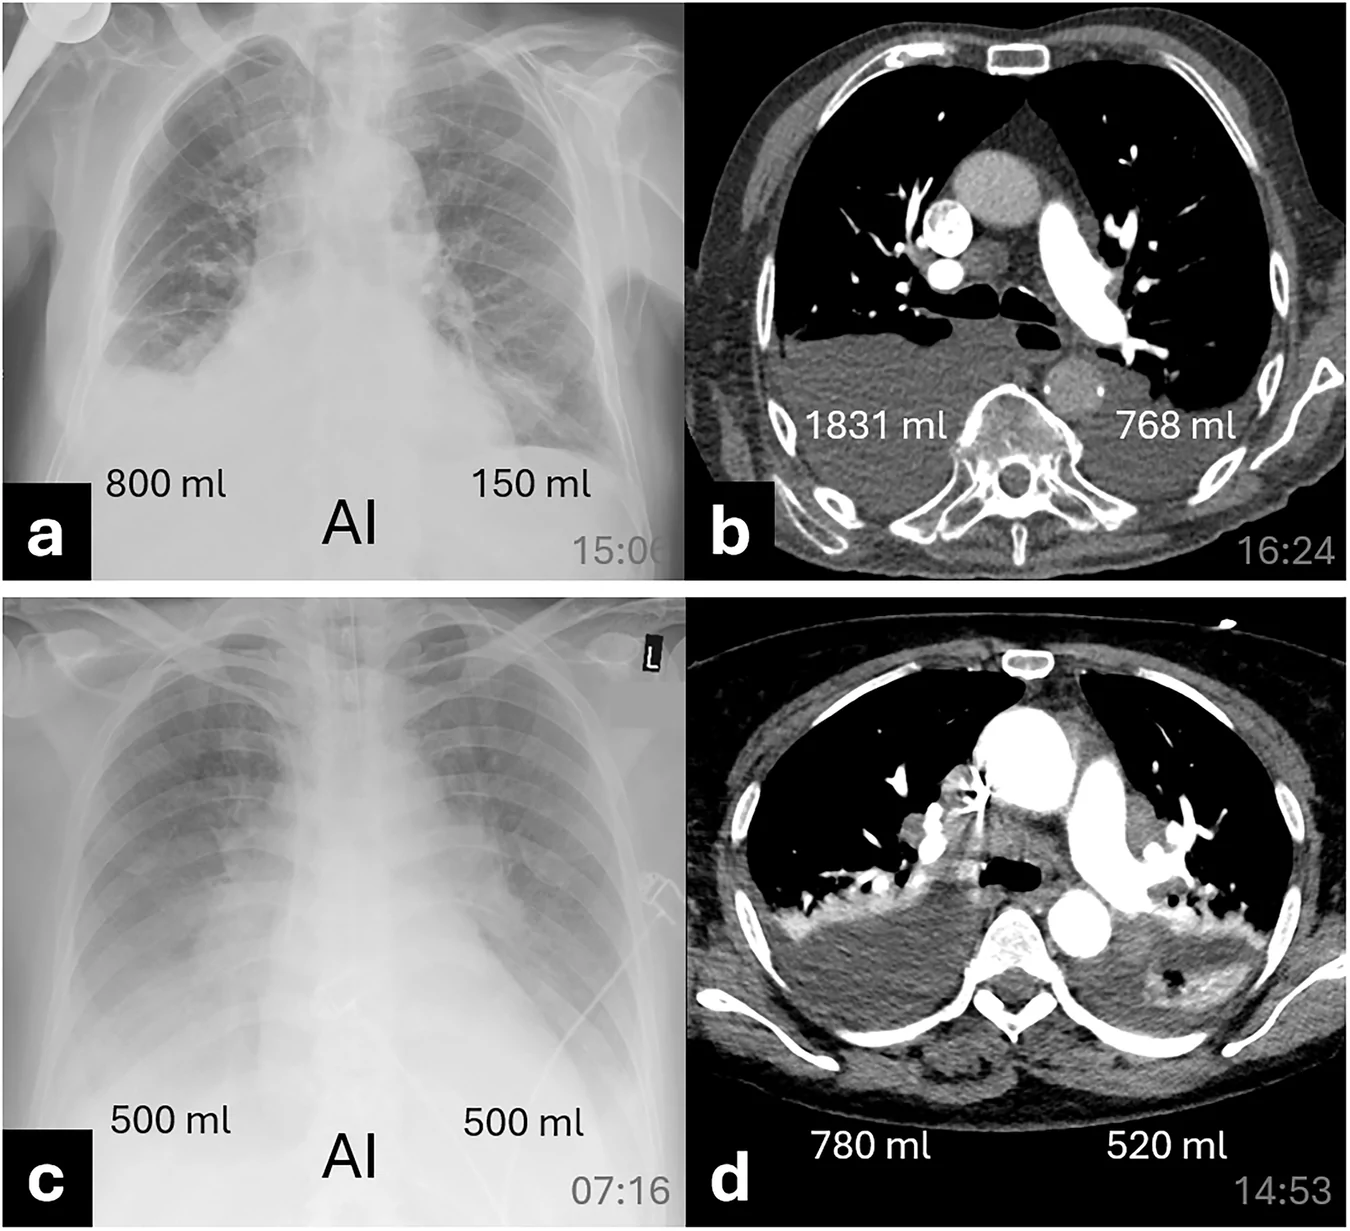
Underestimation of pleural fluid volume on erect chest radiography by AI (a) compared with CT volumetry (b) in a 93-year-old patient with increased anteroposterior chest diameter. In contrast, accurate estimation of pleural fluid volume on supine chest radiography by AI (c) compared with CT volumetry (d) in a 52-year-old female patient
